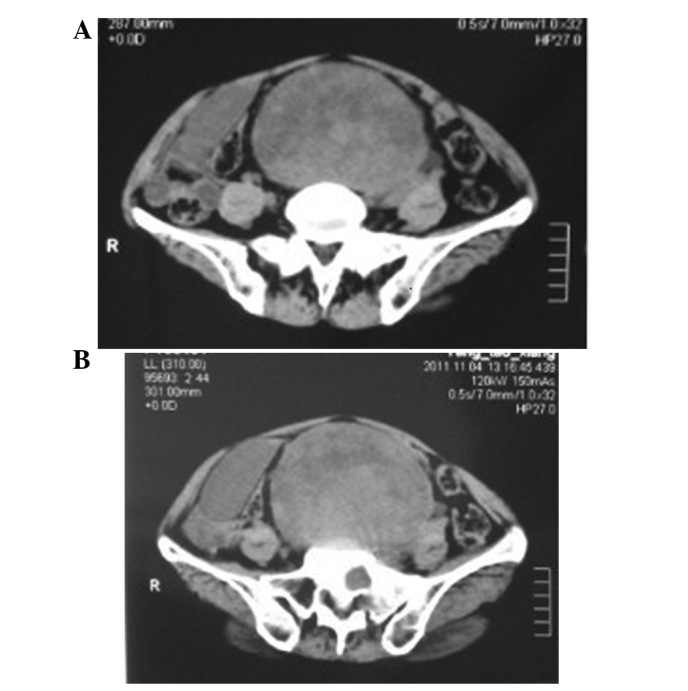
Preoperative CT scan observations. Screening CT showed a giant mass with uneven density originating from the sacral canal without bony destruction. There appeared to be several low-density areas within the tumor and the mass was closely attached to the (A) left ovary and (B) anterior wall of the sacrum. CT, computed tomography.
